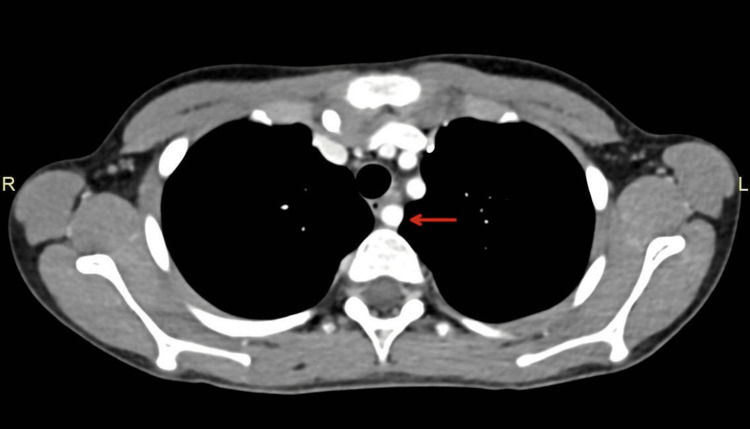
Cross-sectional view of CT angiography revealing an aberrant right subclavian artery (red arrow) coursing retroesophageally.
